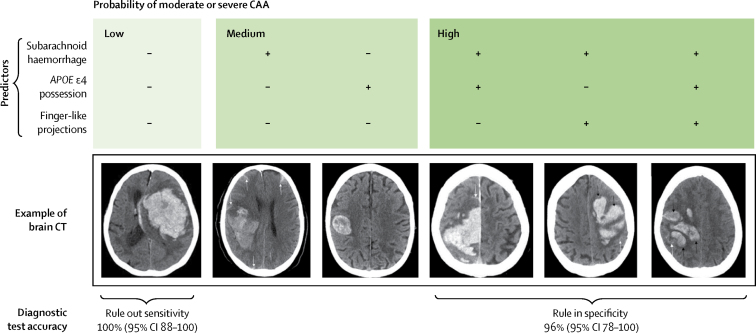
Categorisation of probability of lobar intracerebral haemorrhage associated with moderate or severe cerebral amyloid angiopathy according to the three predictor variables, with example CT images CAA=cerebral amyloid angiopathy. Adapted from Salman and Rodrigues (Creative Commons 4.0).^23^
